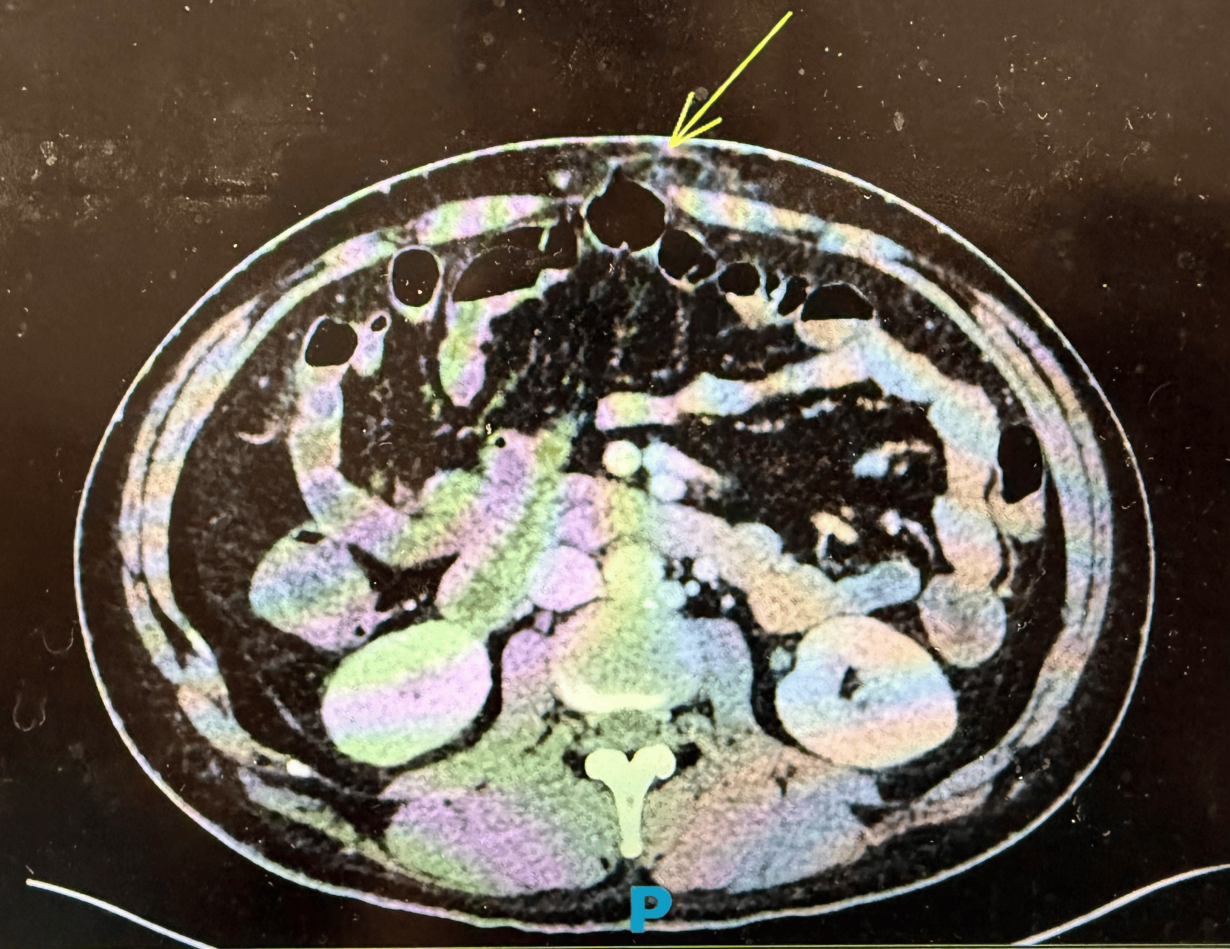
Contrast-enhanced CT of the abdomen showing an epigastric hernia without obstruction (yellow arrow).
